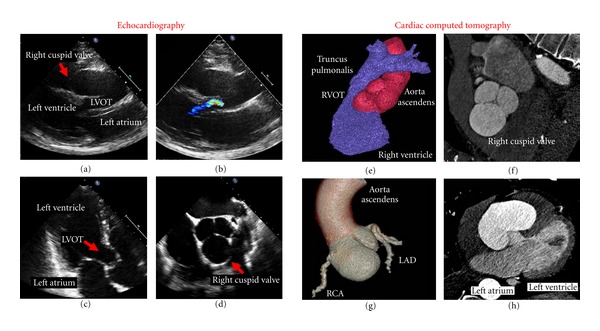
Comparison of transthoracic echocardiography and cardiac computed tomography angiography (CCTA). Short parasternal axis with and without aortic outflow tract (a) and (b), two-chamber view with left ventricular outflow tract (c), and top view with tricuspid aortic valve (d) and (f). Compression of the right ventricular outflow tract (e), 3D reconstruction of the aortic root (g), and four-chamber view (h) in CCTA. (LVOT: left ventricle outflow tract, RVOT: right ventricle outflow tract, LAD: left anterior descending, RCA: right coronary artery.)
